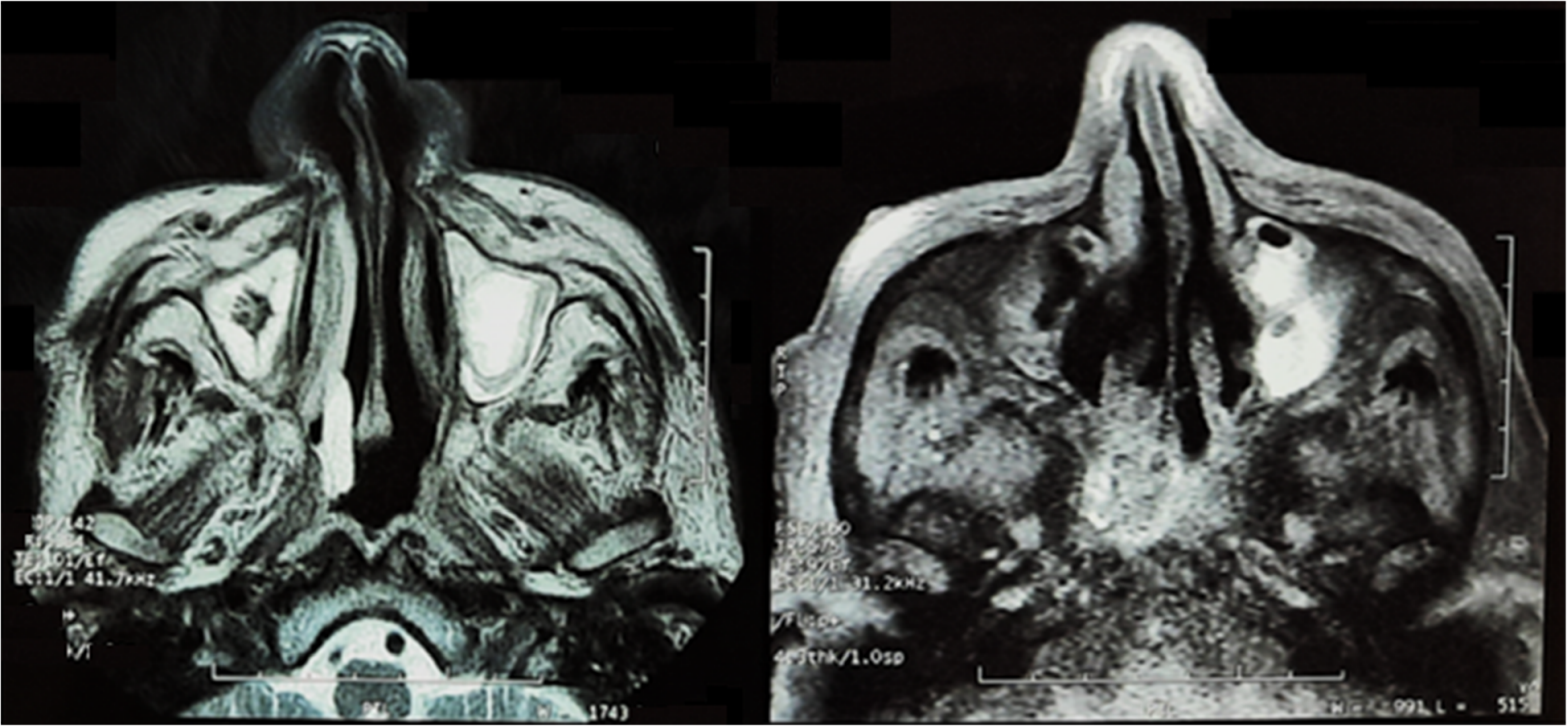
Axial MRI without contrast along the maxillary sinus showing bilateral sinusitis yet more on the left side with hypo intense margins that goes radiologically with fungal infection with heavy metals deposition and hyper intense center that represents bacterial nature
